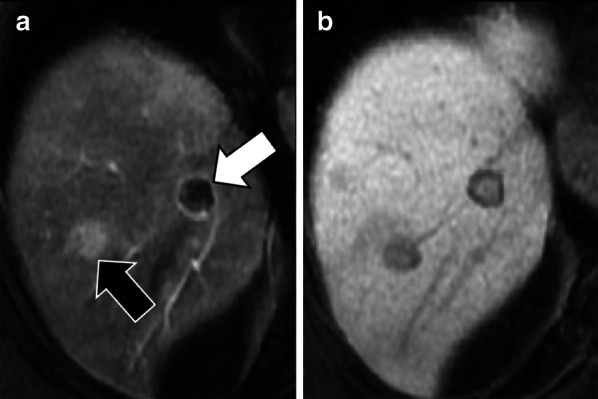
A 73-year-old man with colon cancer and liver metastases. Gadobenate dimeglumine-enhanced MRI in (a) arterial phase and (b) hepatobiliary phase demonstrates two liver metastases with different signal characteristics. A metastasis (black arrow) shows homogenous arterial phase hyperenhancement with a peripheral hypointense rim in the hepatobiliary phase. A second metastasis (white arrow) shows peripheral rim enhancement with a peripheral hypointense rim and cloud-like central enhancement in the hepatobiliary phase
